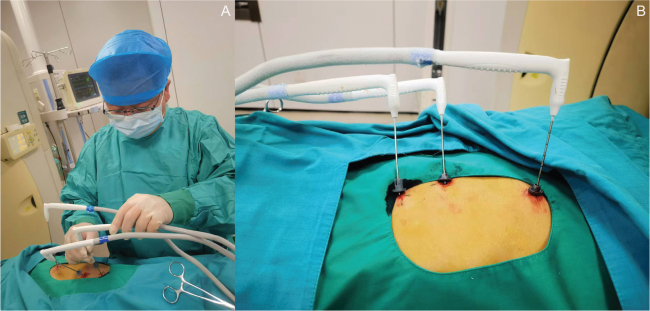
Representative Images of the Argon–Helium Cryoablation Procedure. (A) The physician performing CT-guided percutaneous puncture and insertion of cryoprobes into the tumor. (B) Multiple cryoprobes inserted into the target lesion during the freezing phase of cryoablation, aiming to create an ice ball that encompasses the tumor and a margin.
